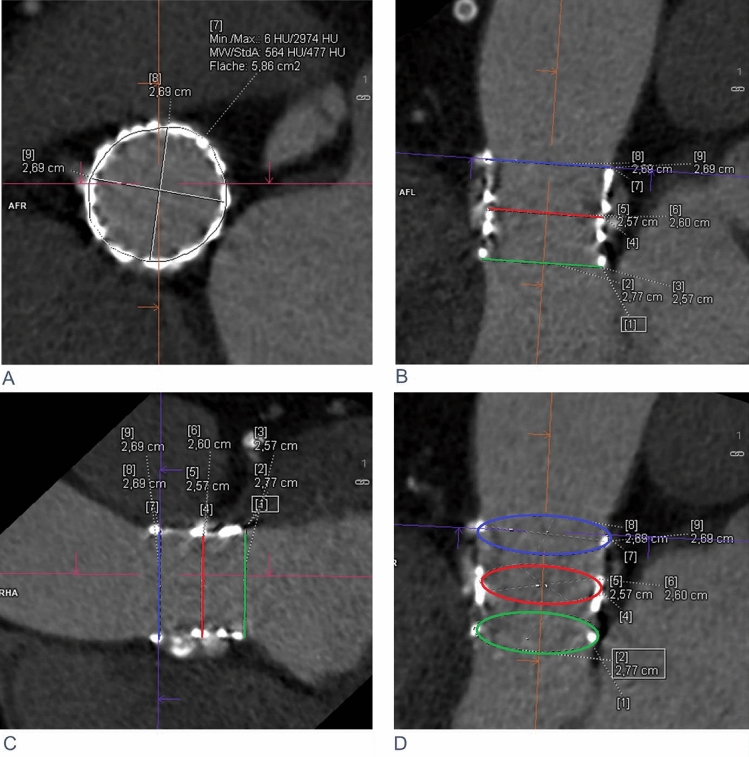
Post-TAVI-CTA measurements. The figure shows the post-TAVI-CTA measurements of a balloon-expanding prosthesis—the area and minimal and maximal diameter at the LVOT end (inflow, green), the stent center (center, red) and the aortic end (outflow, blue). *CTA* computed tomography angiography, *LVOT* left ventricular outflow tract, *TAVI* trans-catheter aortic valve implantation
